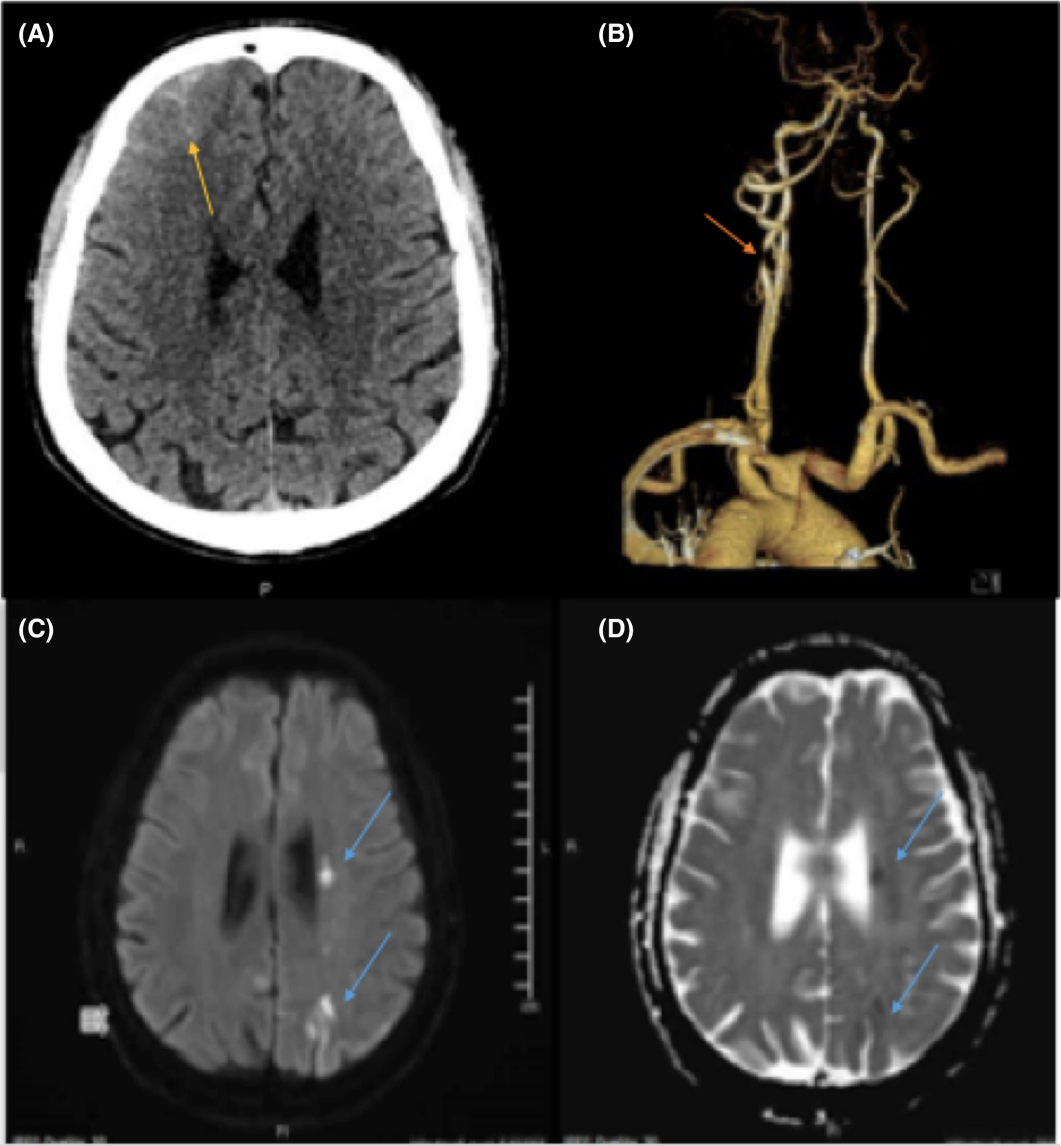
[A–D] Case one with Left ICA stenosis and contralateral cSAH. [A, B] None enhanced CT head: There is minimal subarachnoid hemorrhage along the right frontal sulci. CTA head and neck: There is severe short segment stenosis of the left proximal internal carotid artery. Extensive atheromatous calcification of the cavernous segments of the internal carotid arteries bilaterally. [C, D] MRI, diffusion‐weighted imaging (DWI) and apparent diffusion coefficient (ADC) multiple areas of watershed acute infarcts in the left hemisphere, with contralateral right frontal minimal subarachnoid hemorrhage
